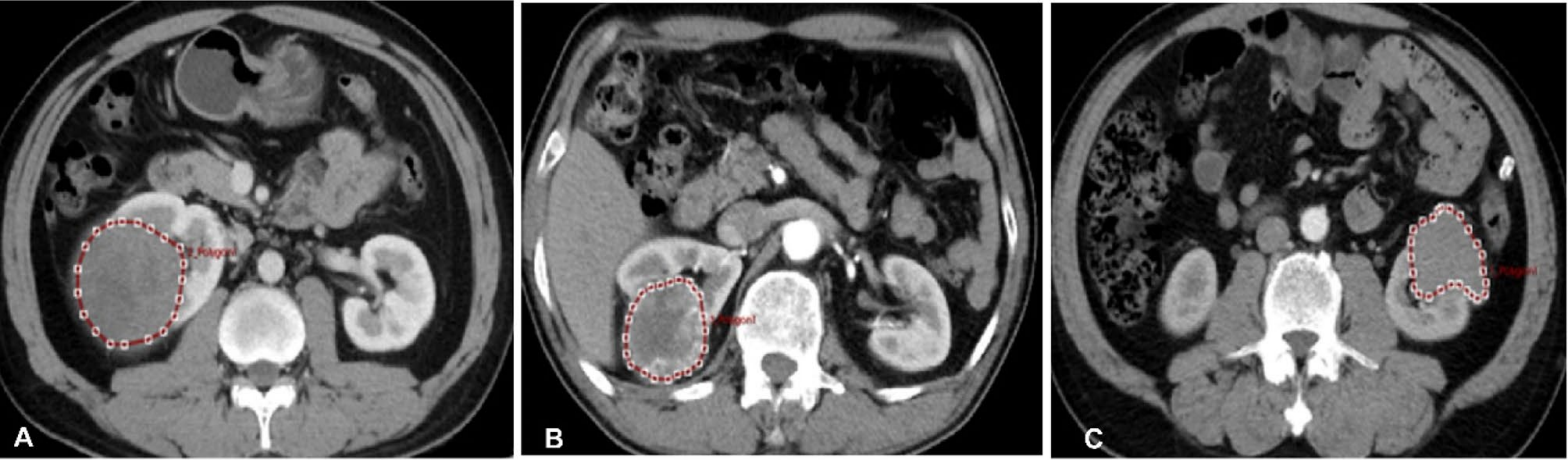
Renal cell carcinoma. (A) A 59-year-old man had cortical phase of papillary renal cell carcinoma (RCC) on computed tomography (CT) enhancement (ROI with dotted line). (B) A 62-year-old woman had cortical phase of clear cell RCC on CT enhancement (ROI with dotted line). (C) A 71-year-old man had cortical phase of chromophobe RCC on CT enhancement (ROI with dotted line).
